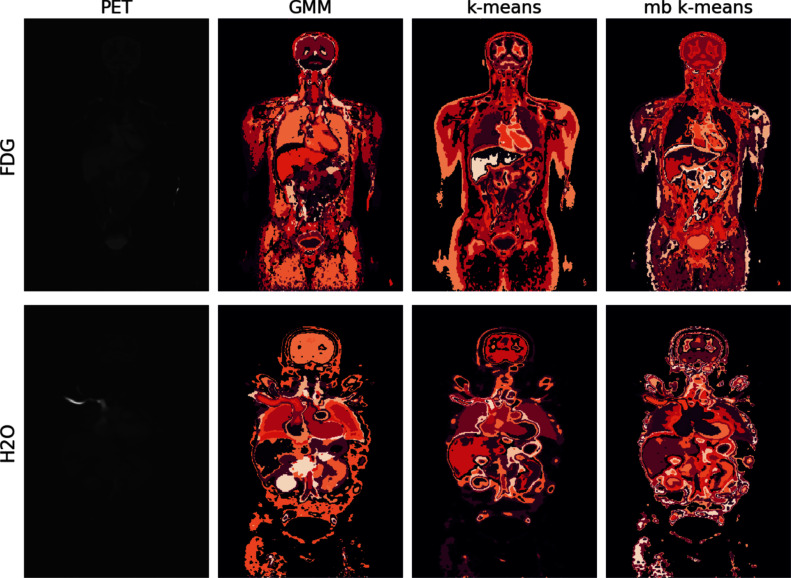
Segmentations of the middle slice of a randomly selected image from the FDG human data (top row) and the H2O human data (bottom row). The left-most panel visualises the corresponding slice of the PET image prior to preprocessing (sum over time points)
